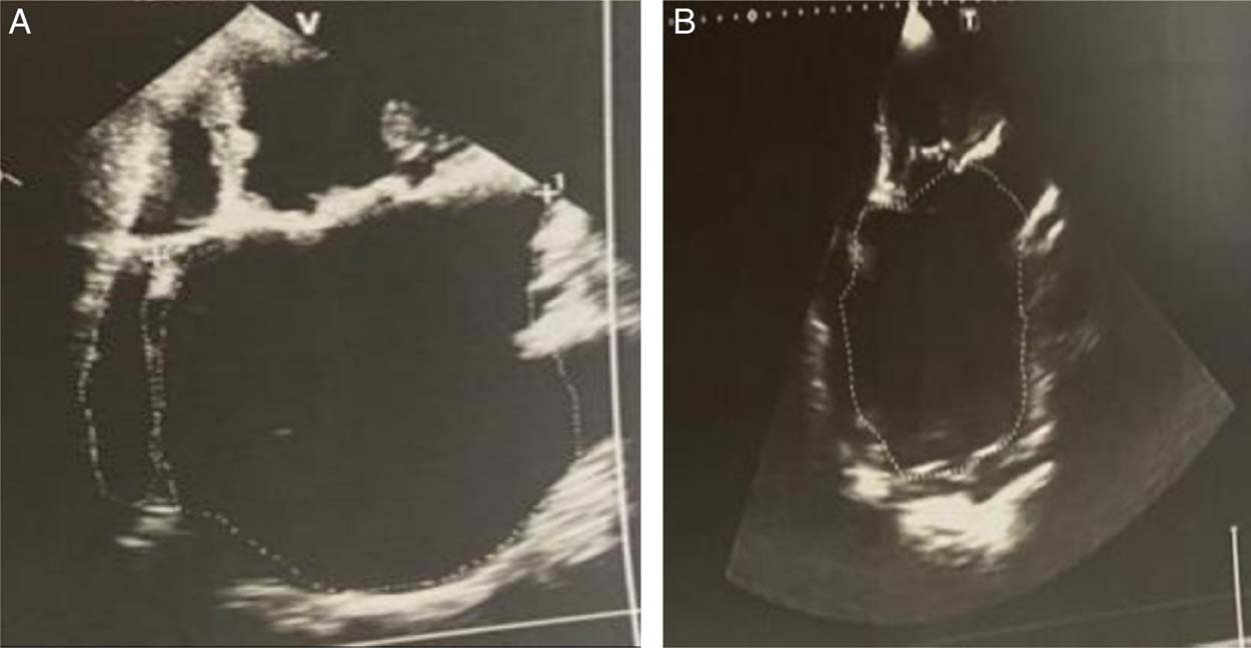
(A) Apical two-chamber window showing a giant left atrium at 130 cm^2^. (B) Apical four-chamber window showing a giant left atrium with a size of 165 cm^2^ in area.
